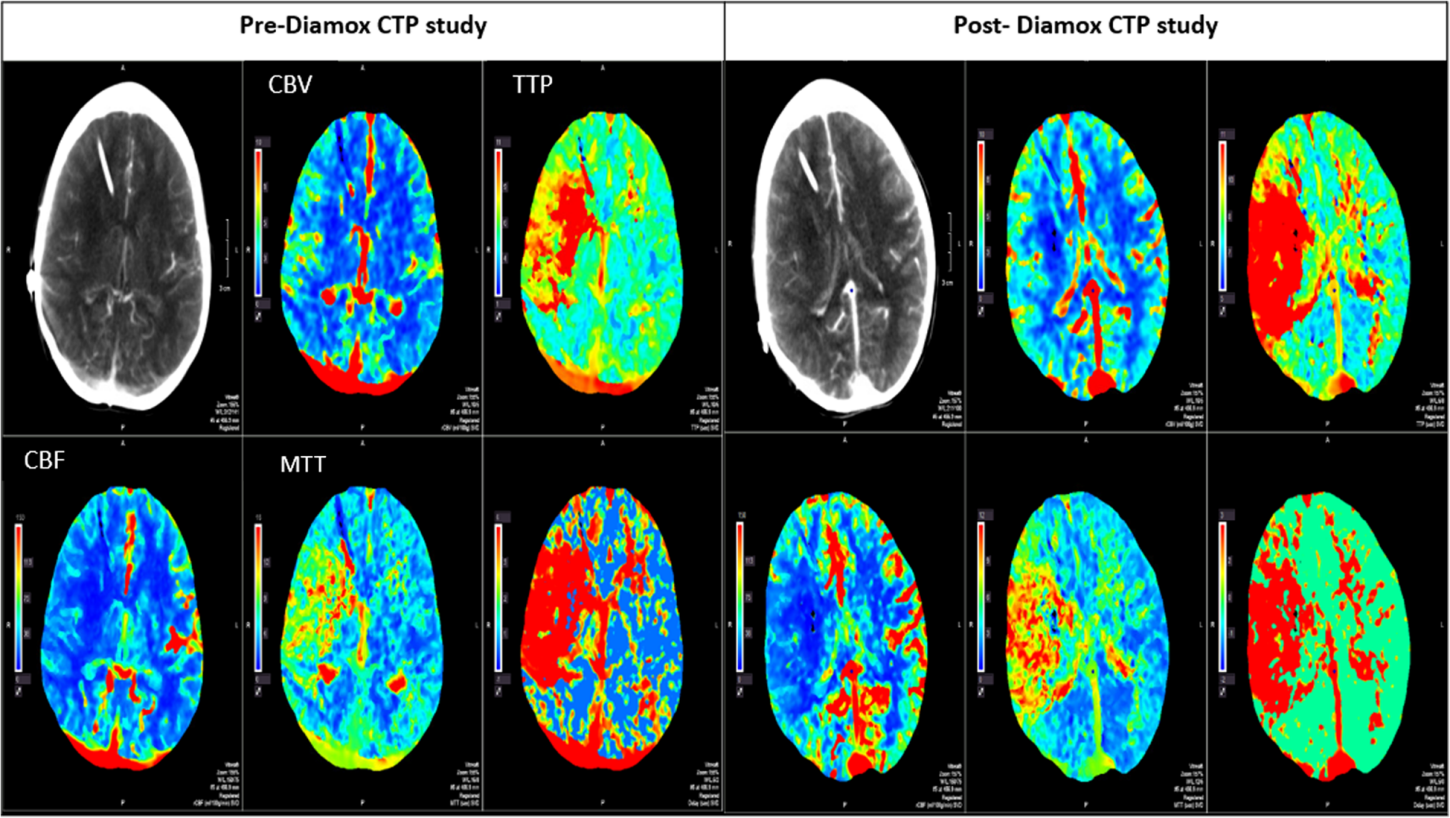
Pre-Diamox computed tomographic perfusion (CTP) study showing a large region of asymmetric diminished cerebral blood flow (CBF) and elevated mean transit time and time to peak within the right middle cerebral artery territory, with few small interspersed regions of decreased cerebral blood volume (CBV). Post-Diamox CTP study showing increased degree of asymmetric decreased CBF and elevated mean transit time (MTT)/time to peak (TTP) abnormality within the portion of the right middle cerebral artery territory, with unchanged region of matched CBV defect
